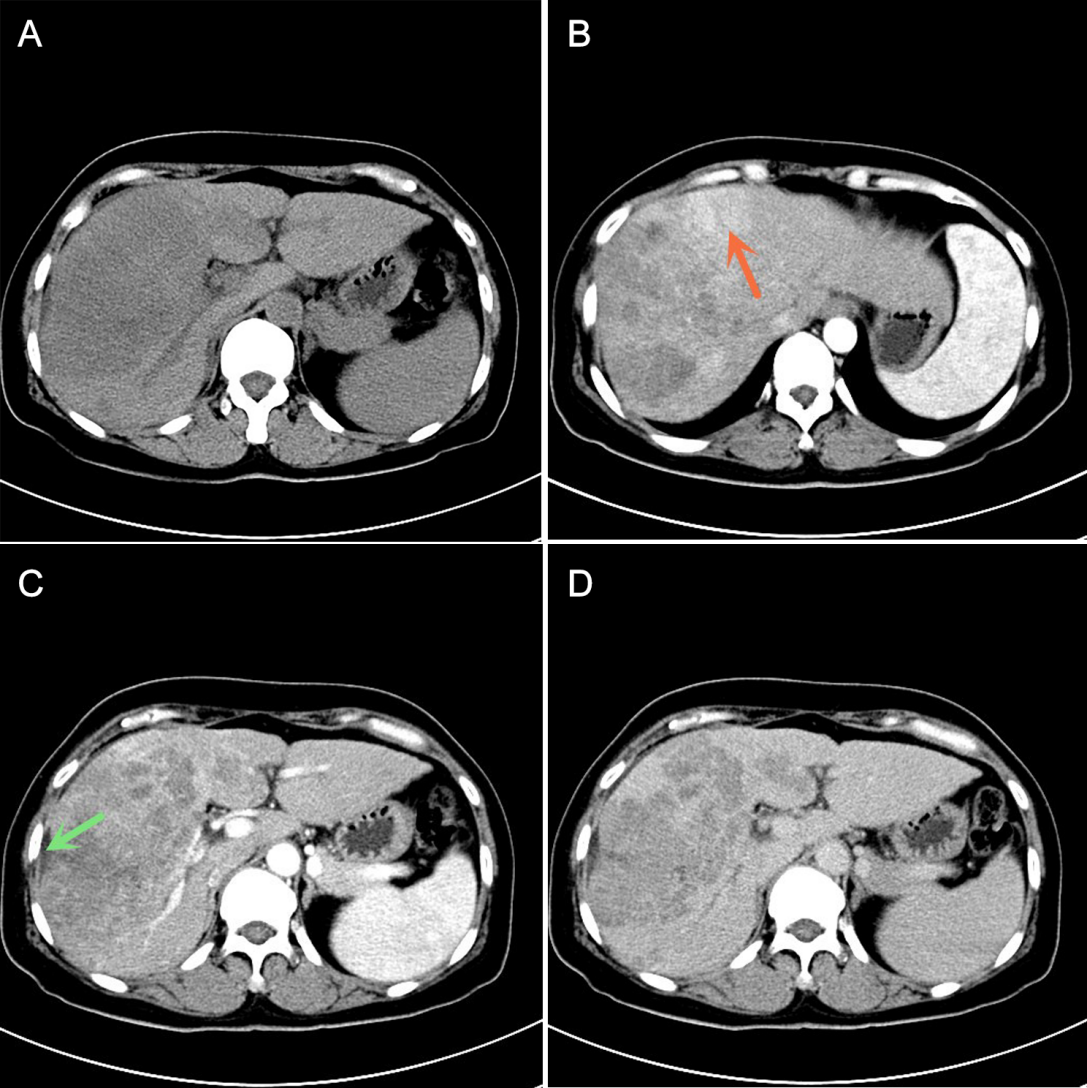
cHCC-ICC presenting with MVI in a 53-year-old woman. (A) A plain CT scan revealed a large low-density mass in the right lobe of the liver, capsule retraction can be seen around the mass (green arrow). (B-D) The arterial phase of the enhanced CT scan showed significantly uneven enhancement and peritumoral enhancement (red arrow)
